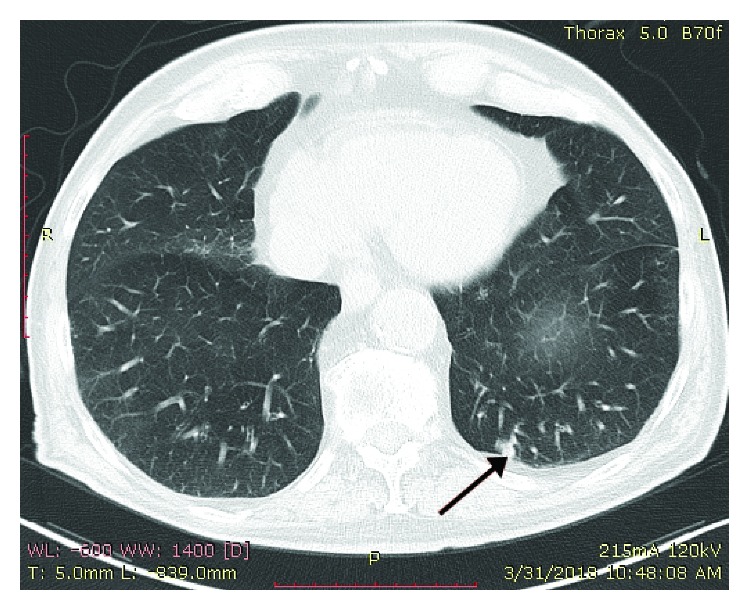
Chest CT image showing small (3 mm) nodules (black arrow) in the apical-posterior segment of the left upper lobe.
